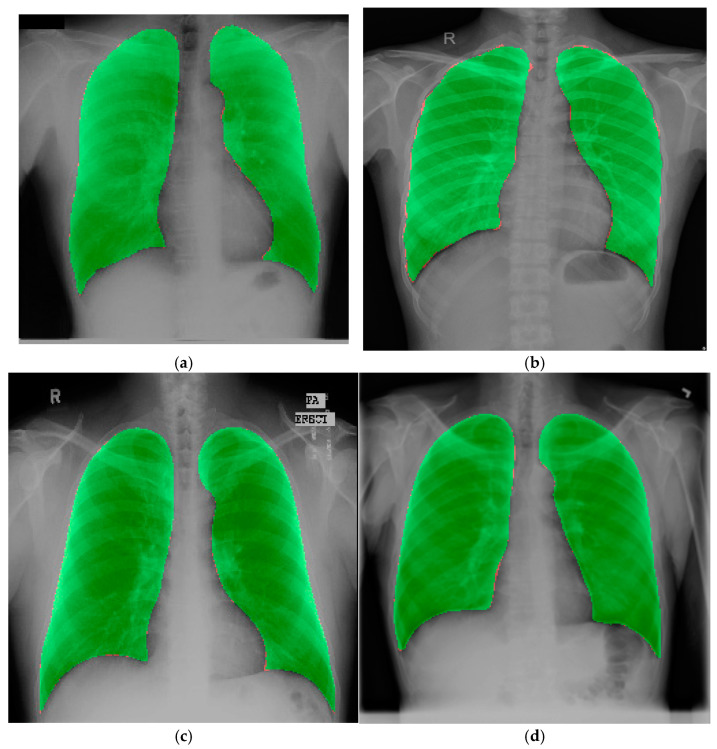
Original mask and proposed model’s predicted mask overlay: (a) JSRT dataset, (b) SZ dataset, (c) MC dataset, (d) Chest X-ray dataset. (The red spot represents the original mask area, and the green spot represents the predicted mask area).
